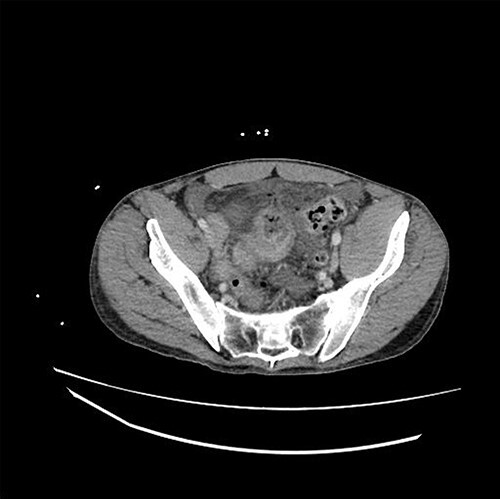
CT demonstrating thickened loop of ileum in the pelvis with pneumoperitoneum and free fluid.
